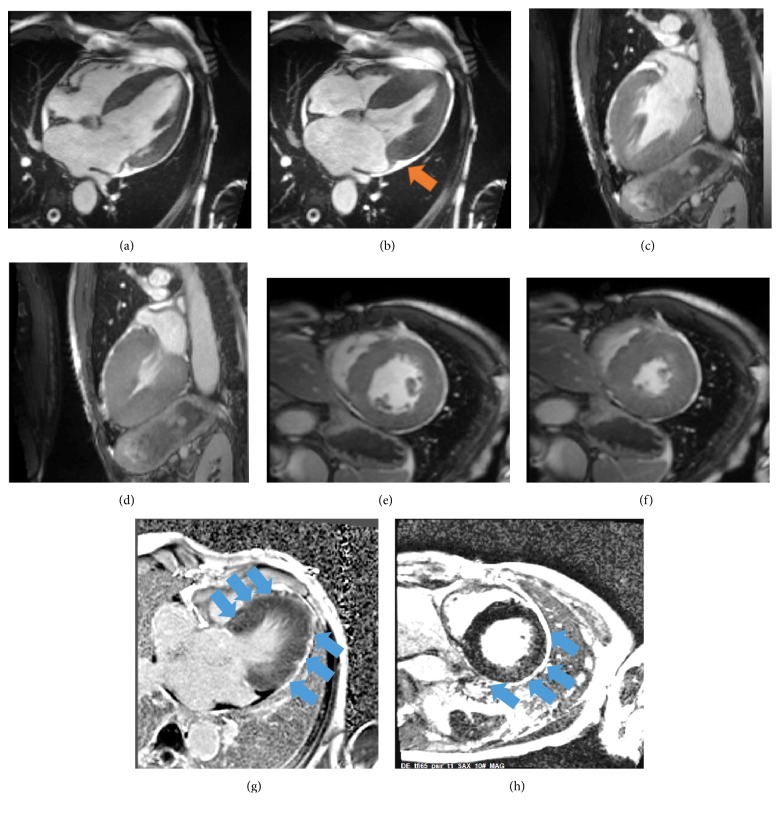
Cardiac magnetic resonance imaging confirmed severe left ventricular hypertrophy and minimal pericardial effusion ((a)–(f), orange arrow in (b)). Late gadolinium enhancement was suspected in corresponding 4-chamber and short axis view images ((g)-(h)).
